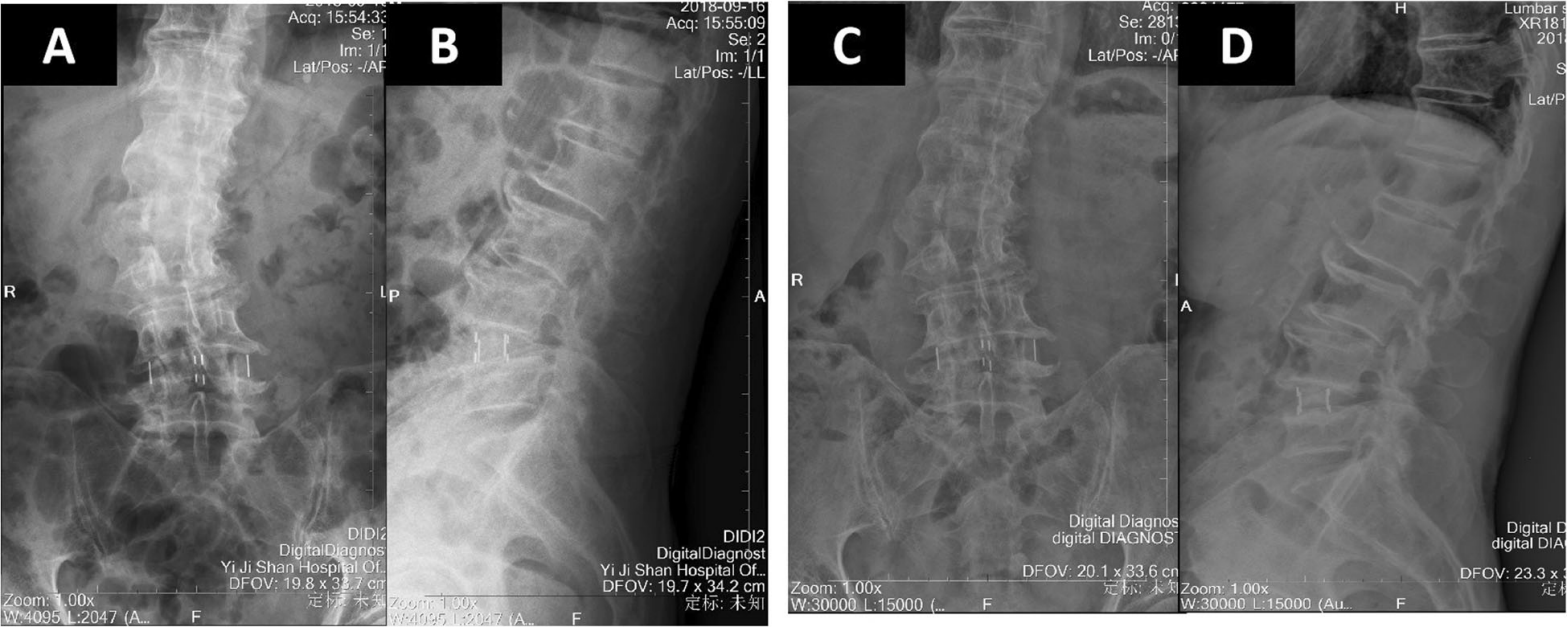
X-ray of the lumbar spine after the stand-alone oblique lateral interbody fusion operation (a, b) and three-month follow-up (c, d)
